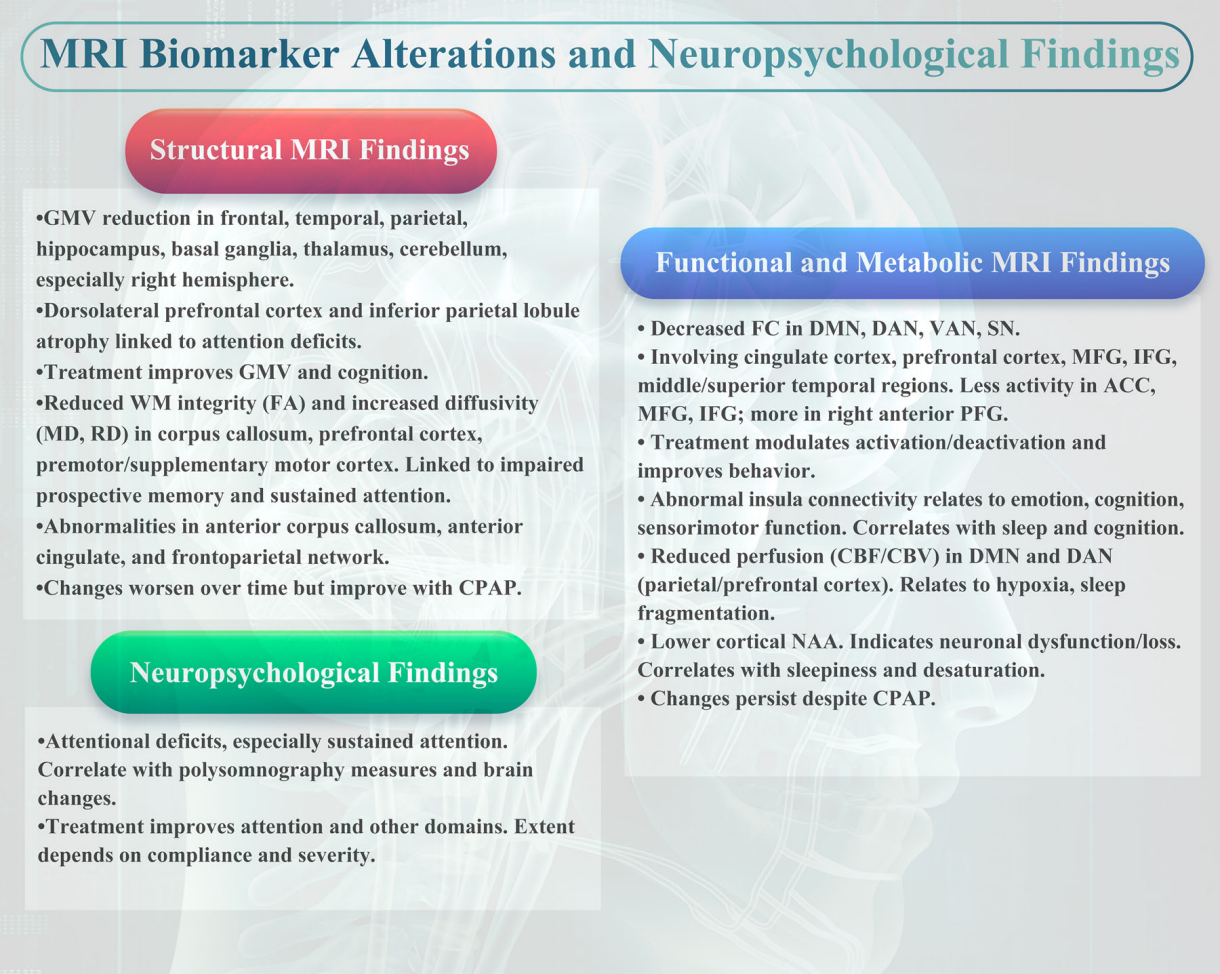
Main magnetic resonance imaging (MRI) biomarker alterations and neuropsychological assessments in obstructive sleep apnea (OSA) patients.
